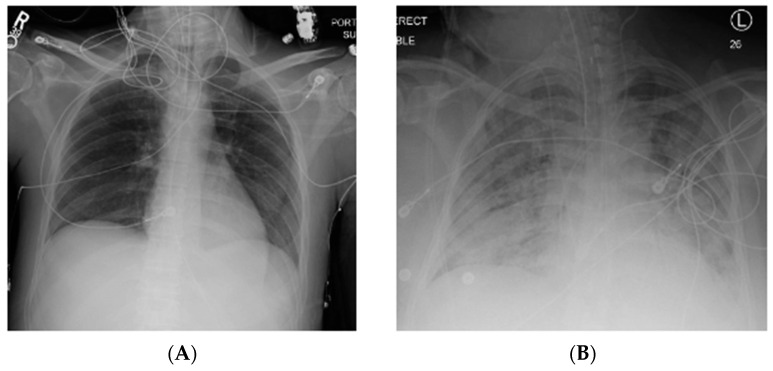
Normal chest X-ray (A) compared to that of a patient with acute respiratory distress syndrome (ARDS) (B) with bilateral pulmonary infiltrates. R, right; L, left.
